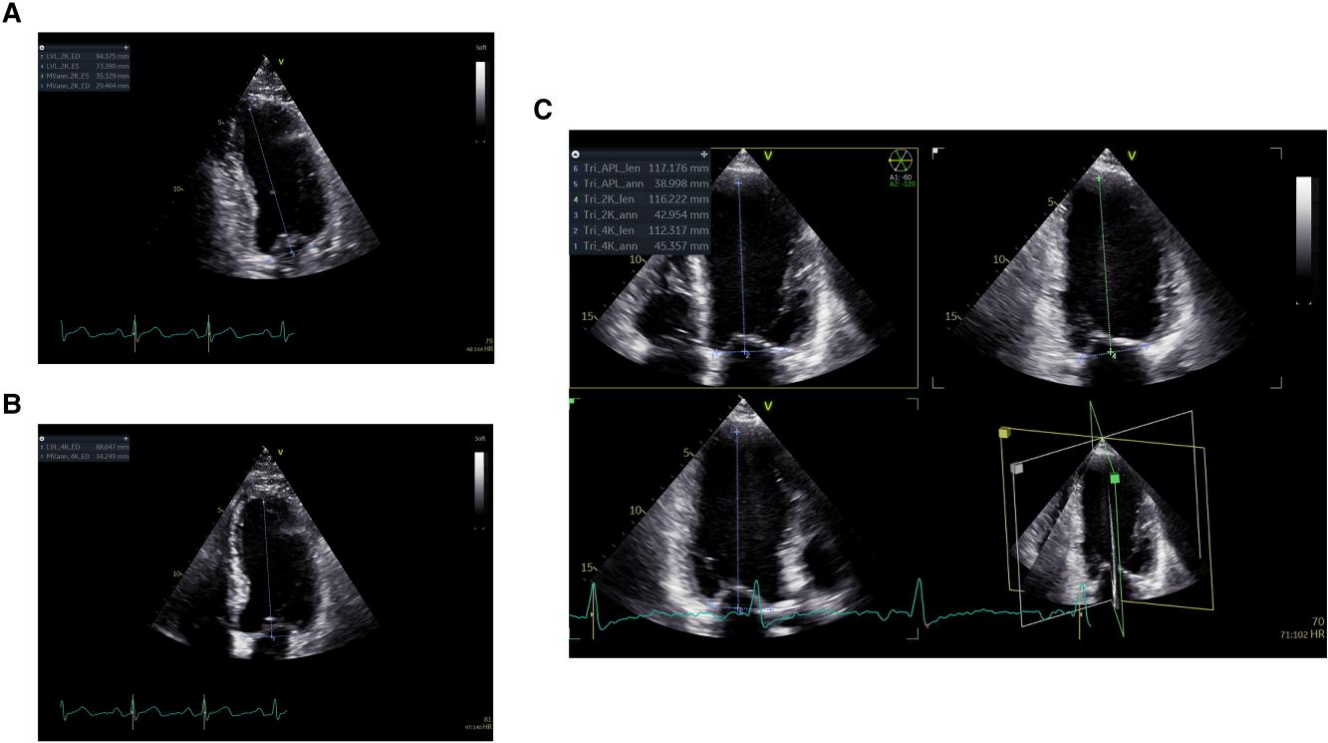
LV length measurement method. Screenshots of calipers used for measuring LV length at the end-diastole. First, a caliper is placed between the two mitral annular points. Secondly, the LV length is estimated by placing another caliper from the subendocardial apical point to the centrum of the first mitral annular caliper. (*A*) Apical two-chamber recording. (*B*) Apical four-chamber recording. (*C*) Cardiologist’s tri-plane recording used for reference LV length. The mean LV length of the three planes was used as reference. The minor differences between views are partly explained by mispositioning of the apical point in four-chamber view, while the mitral annular curvature may also explain some variability between views.
